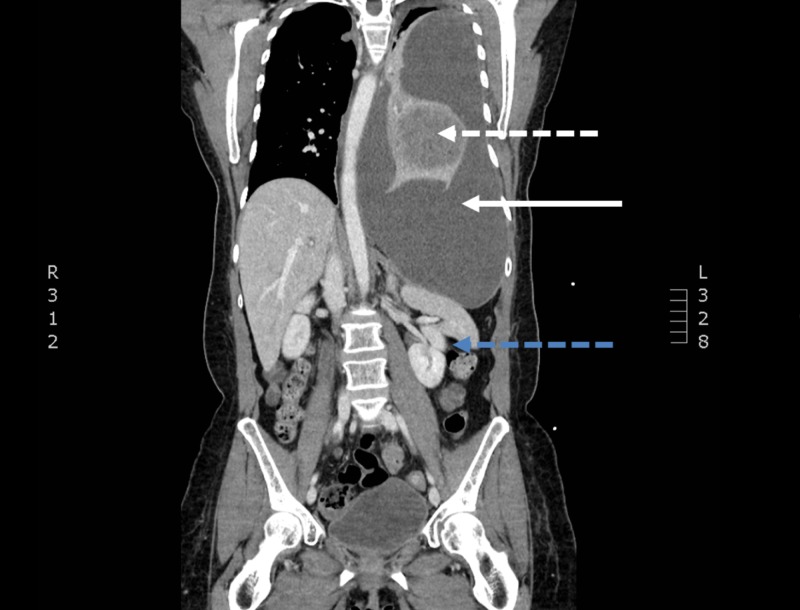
A coronal slice of contrasted computed tomography of the chest, abdomen, and pelvis demonstrating a complex mass (dashed white line) with associated large left-sided pleural effusion (solid white line) with mass effect on the left hemidiaphragm, left kidney, and spleen (dashed blue line)
